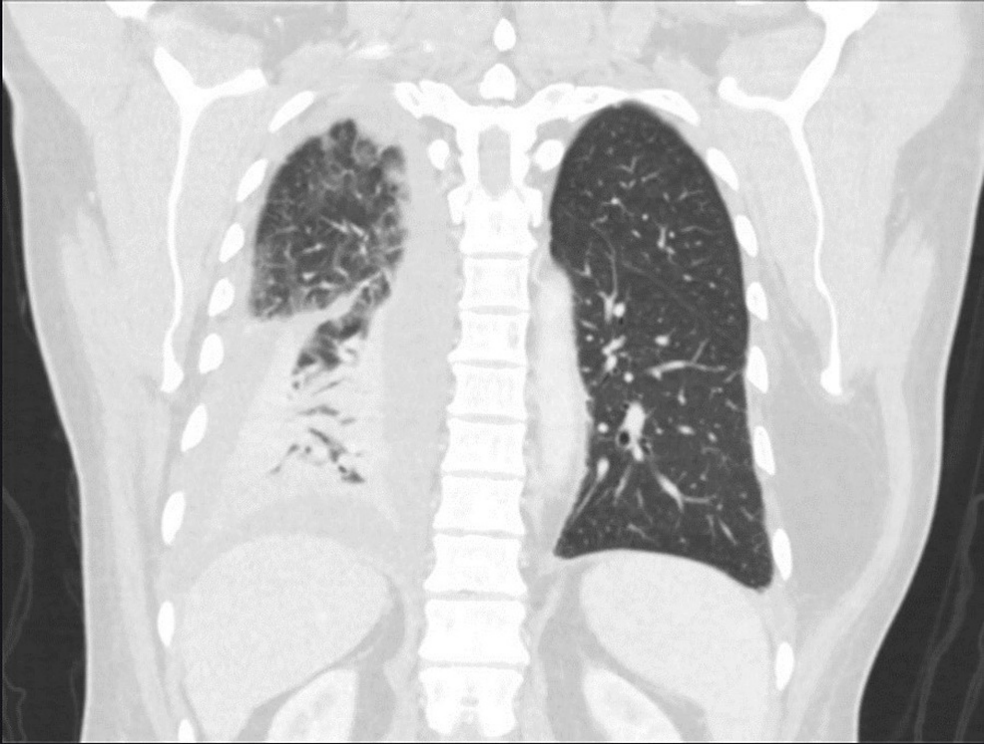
CT chest showing right lower lobe pneumonia with associated moderate-sized pleural effusion
